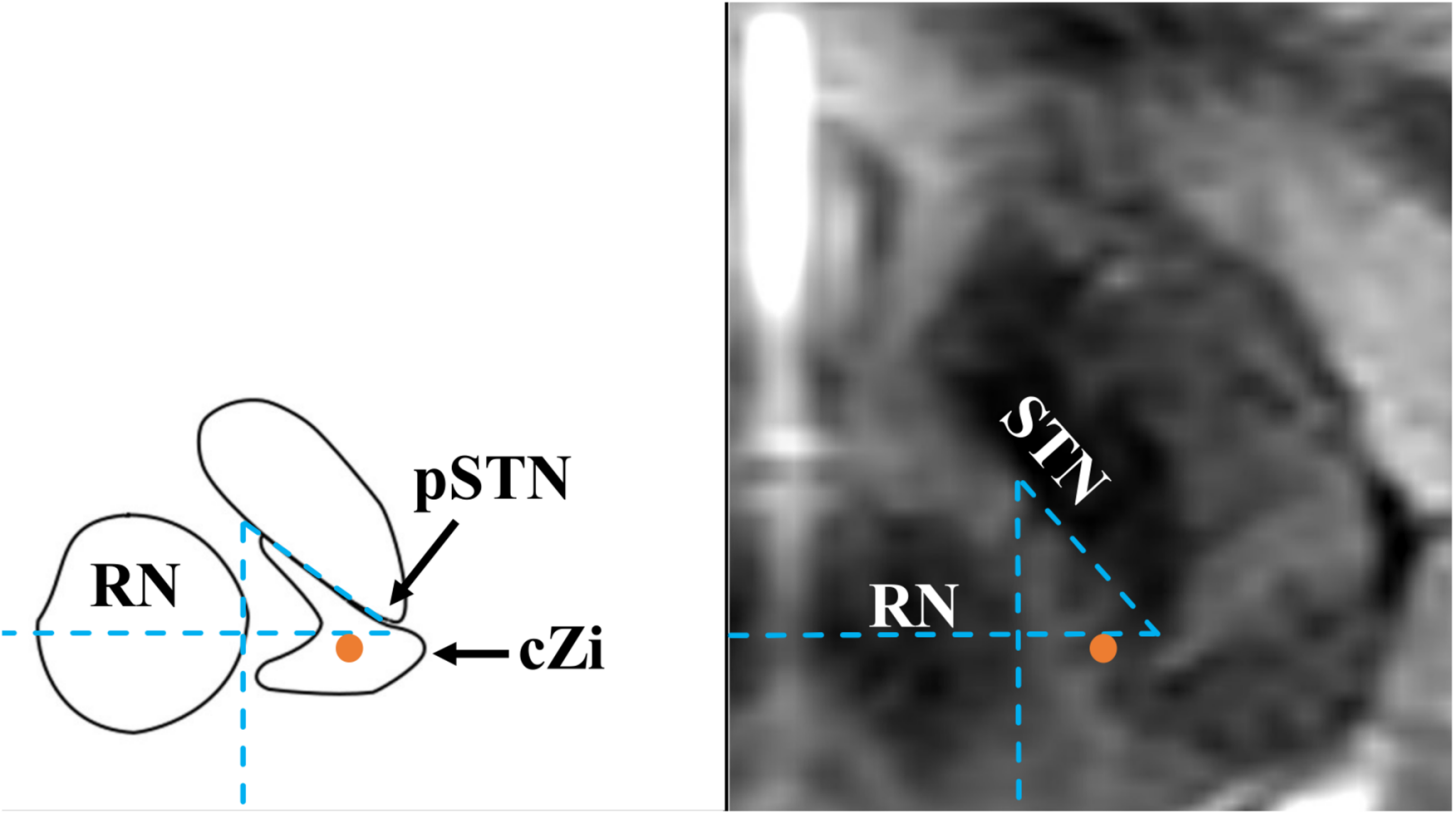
The target (orange marker) with mental helplines. As the relation between the red nucleus (RN) and the posterior tail of the subthalamic nucleus (STN) varies from atlas to MRI, as well as between patients, the target marker has been placed in a position, which would be deemed as adequate lead placement. To the left: the location and size of the caudal zona incerta (cZi) on the level of Z = -3.5 mm. Based on the Atlas for Stereotaxy of the Human Brain by G.Schaltenbrand and W.Wahren, 1977 © Thieme. To the right: T2-weighted MRI at the level of the maximum diameter of RN.
